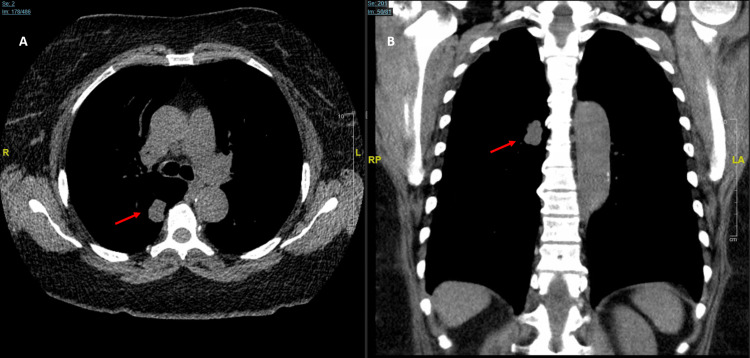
Interval CT chest (axial and coronal views) on the ion robotic protocol obtained prior to bronchoscopy. CT chest (axial and coronal views) obtained prior to robotic bronchoscopy; the images demonstrate significant interval growth of the right lower lobe mass (red arrows) from 1.6 cm to 2.2 x 1.4 cm over a six-month period, highlighting the rapid tumor kinetics characteristic of high-grade neuroendocrine carcinoma.
